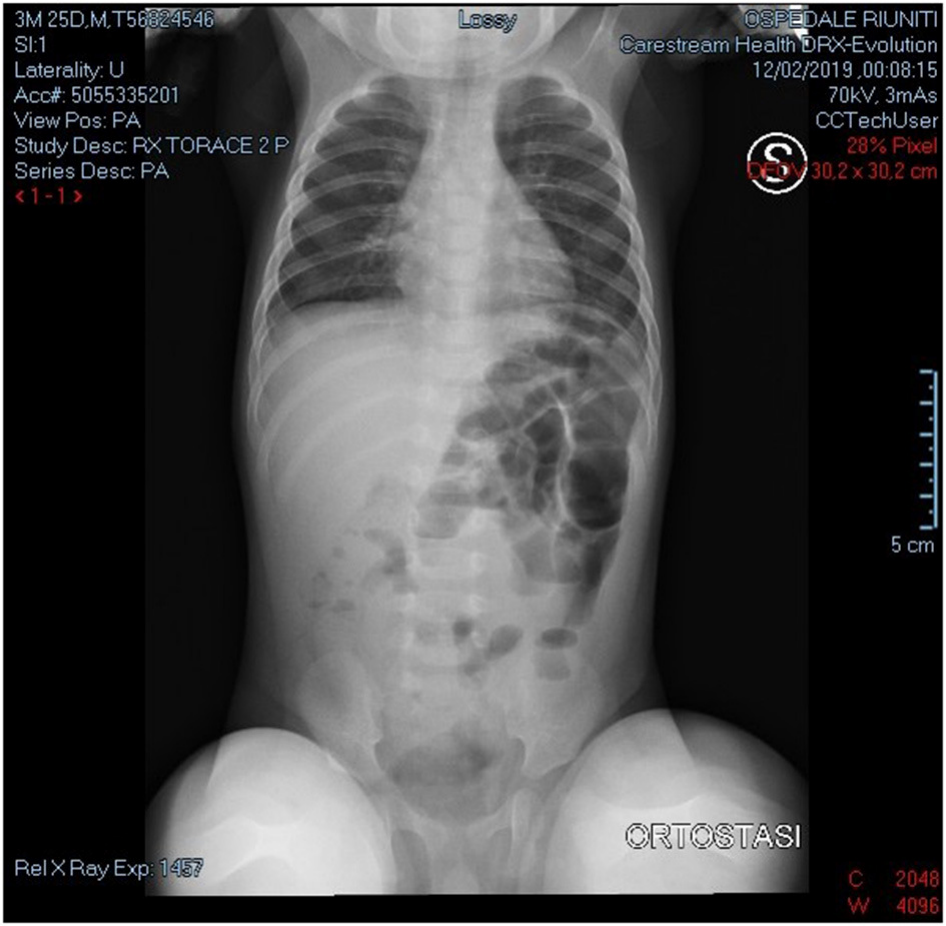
Plain abdominal x-ray showed air fluid levels.
